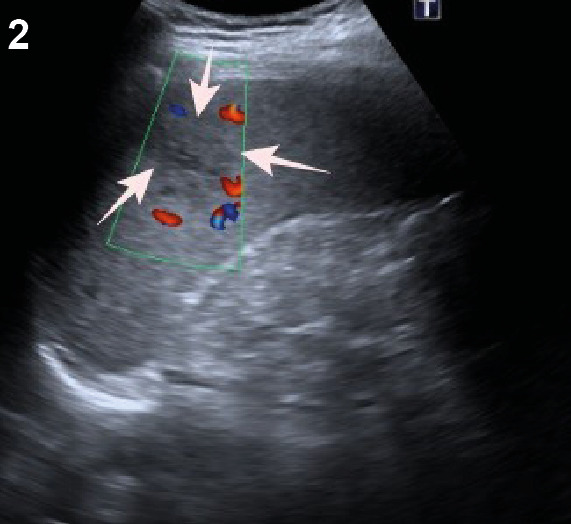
Ultrasonography showing a patchy hypoechoic area in the spleen (white arrows).
